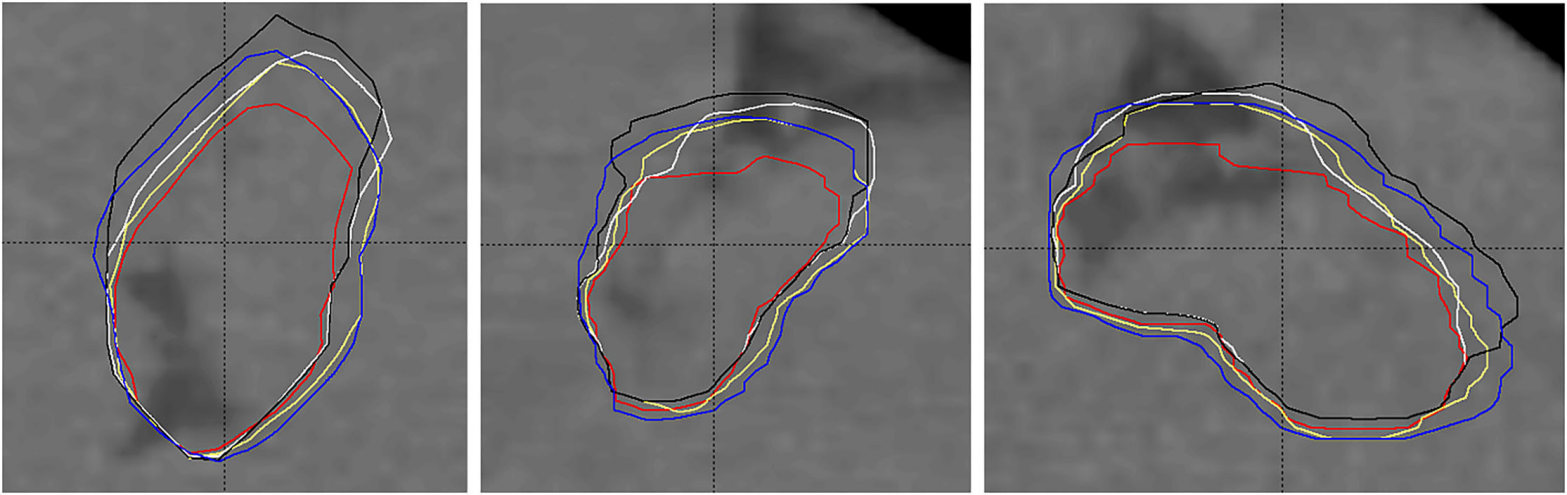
Example of planning target volumes: original treated PTV (red, smallest), PTV_GDM_ (blue), PTV_GDM−SUM_ (yellow), PTV_MIM_ (white), and PTV_VELO_ (black). 1) RefSTRUCT (LMCA) on background CT image used as target surrogate, 2) anisotropic extension of the original target toward inspiration, 3) lesser differences among GDM- and DIR-based volumes than their difference to the original PTV.
